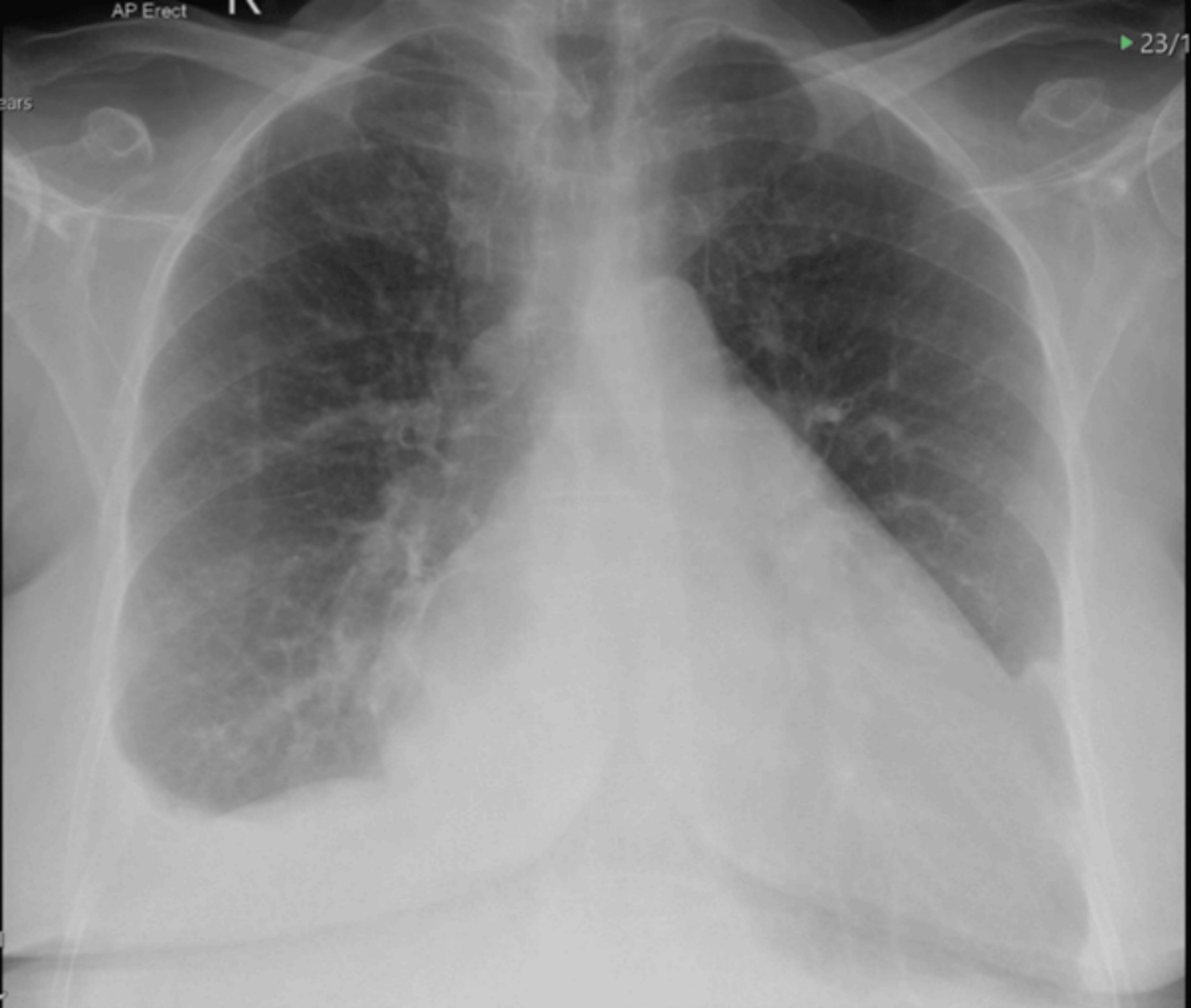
Anteroposterior chest radiograph showing cardiomegaly and bilateral pleural effusions.
